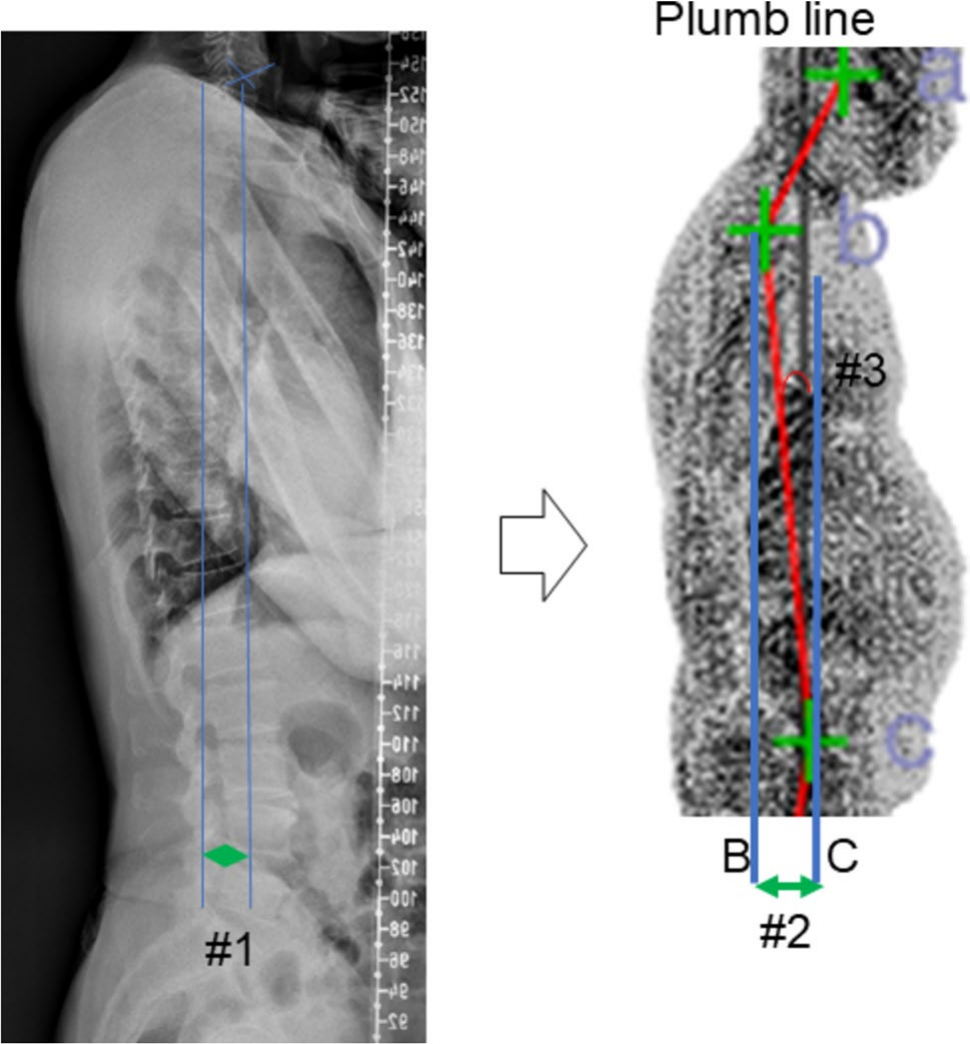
#1. SVA on x-ray / #2. dBC_hor / #3. aBC_sag on the 3D scanner
